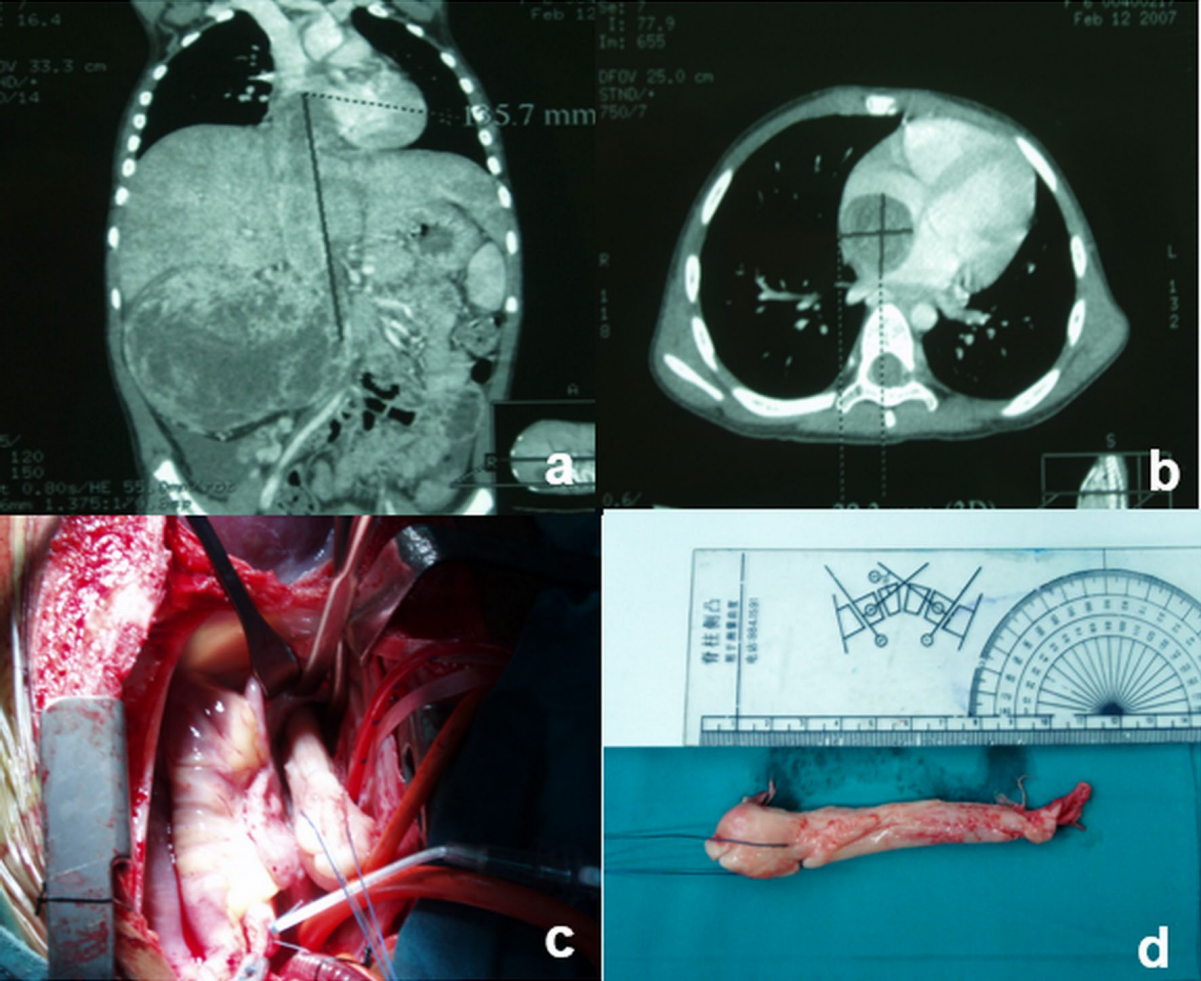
The patient (no. 8 in Table 1), female, was 4.8 years old, with right renal Wilms tumor combined with atrial tumor thrombus. She underwent deep hypothermic cardiopulmonary bypass to remove intra-cardiac tumor thrombus. a, b computed tomography showed right renal tumor and atrial tumor thrombus before preoperative chemotherapy. The tumor shrank after chemotherapy, but the intra-atrial tumor thrombus did not shrink significantly; c, d intra-atrial tumor thrombus was removed under cardiopulmonary bypass in the operation
